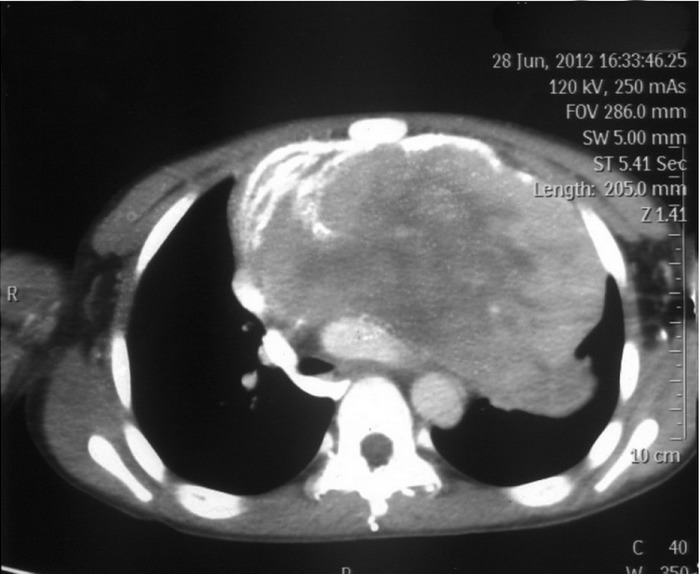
CT scan showing a large mass, 14.1×6.7 cm^2^ in size, in the anterior mediastinum. CT, computed tomography.
